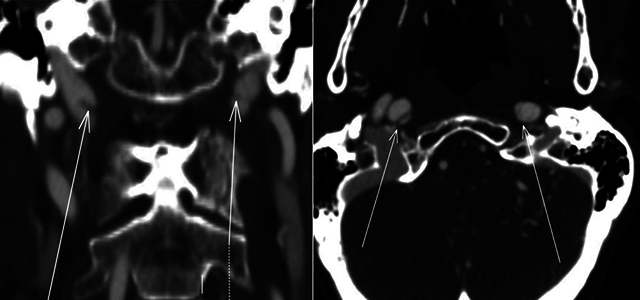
CT angiography showing sequelae of dissection in the cervical portion of the bilateral internal carotid arteries, with an intimal flap indicated by an arrow.
